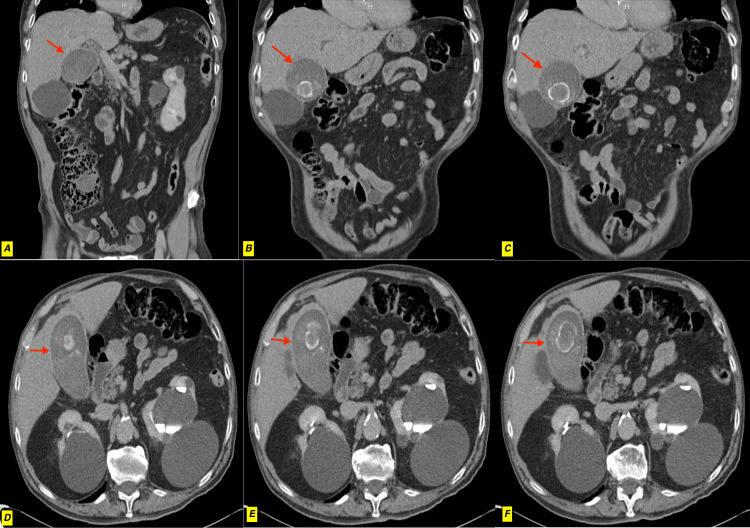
CT abdomen images CT scan of the abdomen showed a distended gallbladder (A) containing gallstones (B, C, D, E, F) and thickening of the gallbladder wall (D, E, F).
